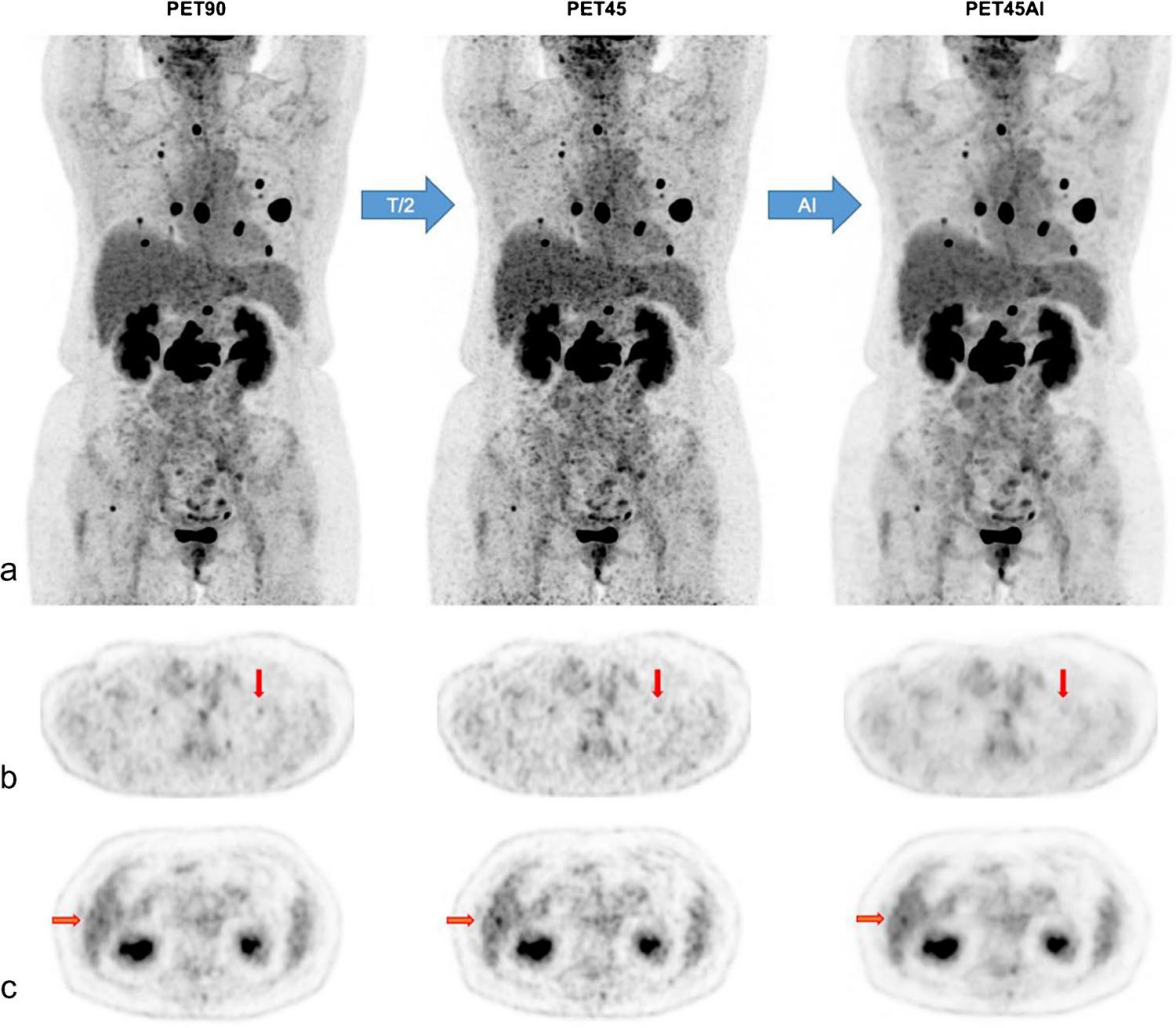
Discordant lesions A 59-year-old women (66 kg; BMI 23 kg/m^2^) with a history of breast cancer showing multiple lung and bone metastases. MIP views (a) and axial PET slices (b and c) of [^18^F]FDG PET90, PET45, and PET45AI. Vertical red arrows in (b) demonstrate one lung metastasis in the upper lobe of the left lung only detected in PET90, measuring 2 × 3 mm on CT with standard SUL_max_ of 1.1 g/ml in PET90. In c a false positive hepatic focus in PET45 (horizontal red arrows)
